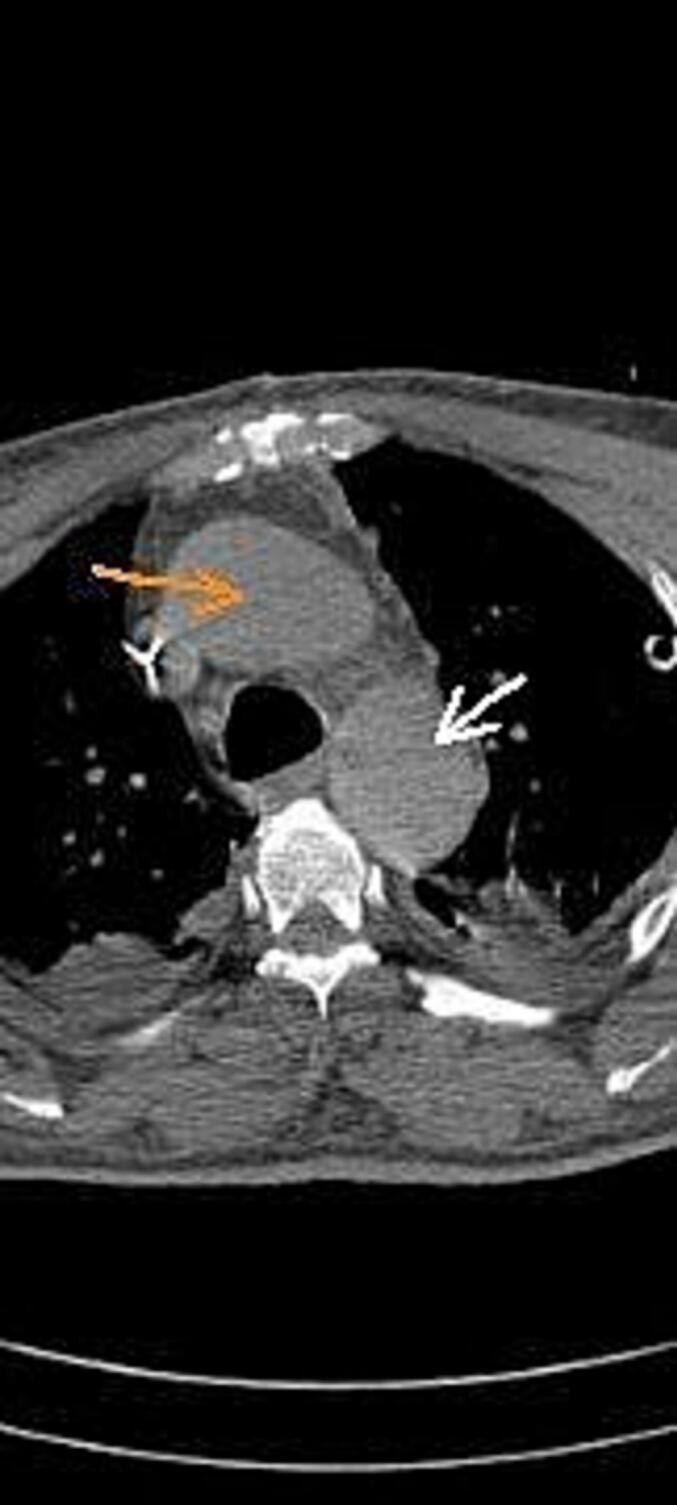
In this CT angiography image, orange arrow indicates dissection in ascending aorta and the white arrow shows dissection in descending aorta. (For interpretation of the references to color in this figure legend, the reader is referred to the web version of this article.)
